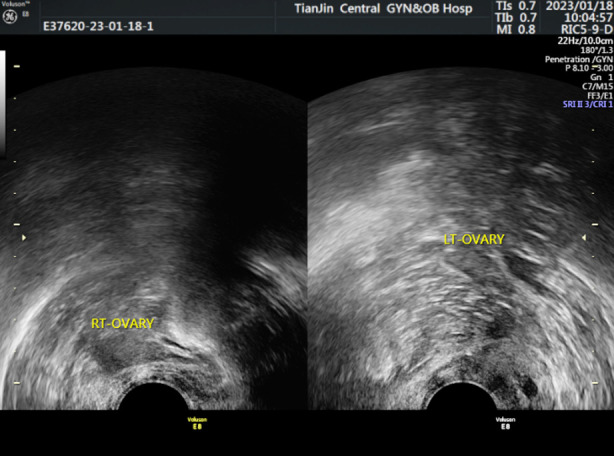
Ultrasonic examination image of POF-group.
